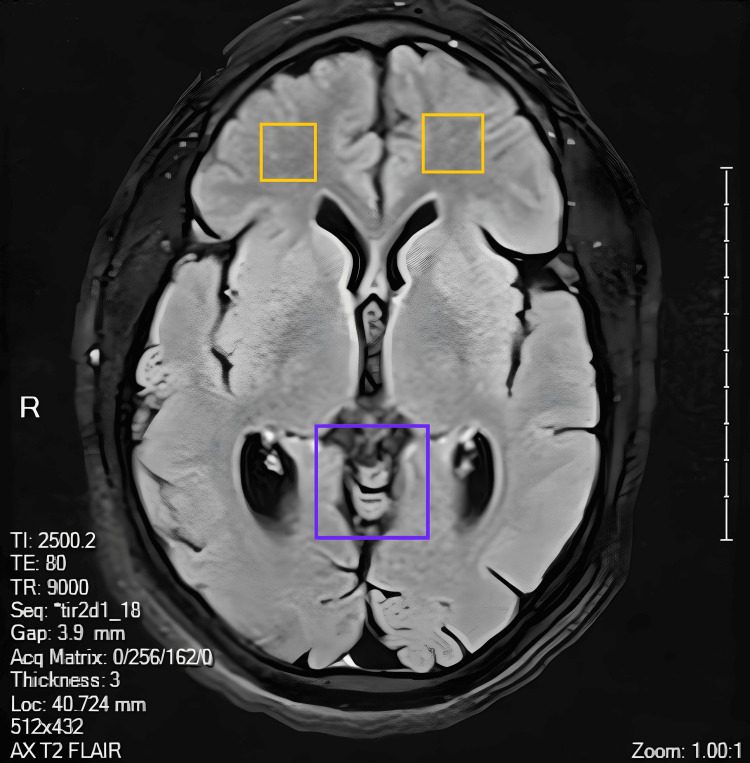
Axial T2/FLAIR MRI. Axial FLAIR MRI, the purple rectangle highlights a CSF-filled space in the posterior periventricular region, corresponding to the structure described posterior to the third ventricle on sagittal imaging. The yellow rectangles show subtle bilateral hyperintensities in the frontal white matter, consistent with nonspecific white matter changes, as described in the radiology report. FLAIR: fluid-attenuated inversion recovery.
